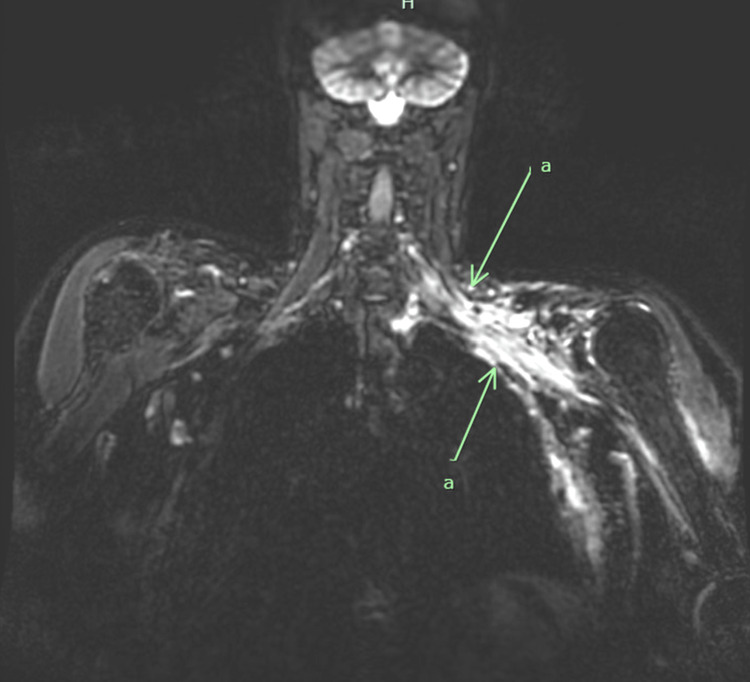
Coronal Chest MRI Without Contrast of Brachial Plexopathy (a) T2 hyperintense signal tracking along the entire course of the left brachial plexus extending from the trunk along the neurovascular bundle to the left axilla
